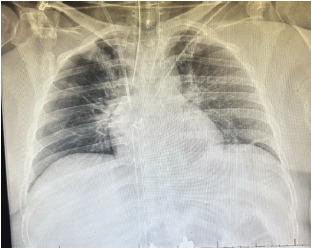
Post-intubation chest radiograph.
